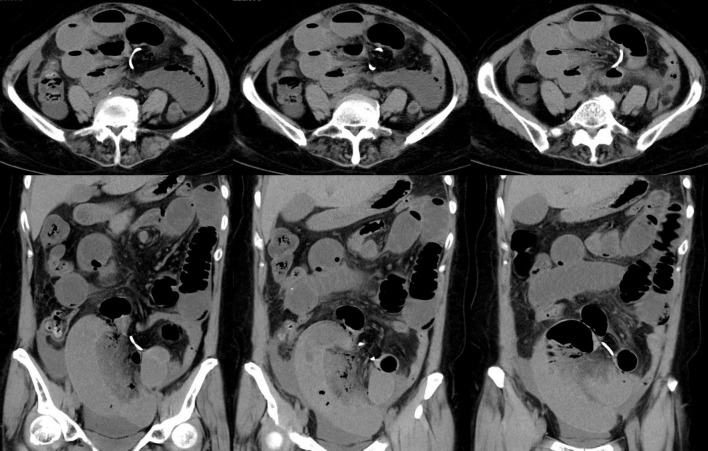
CT imaging demonstrated a ring-shaped high-density shadow in the abdominal cavity.
